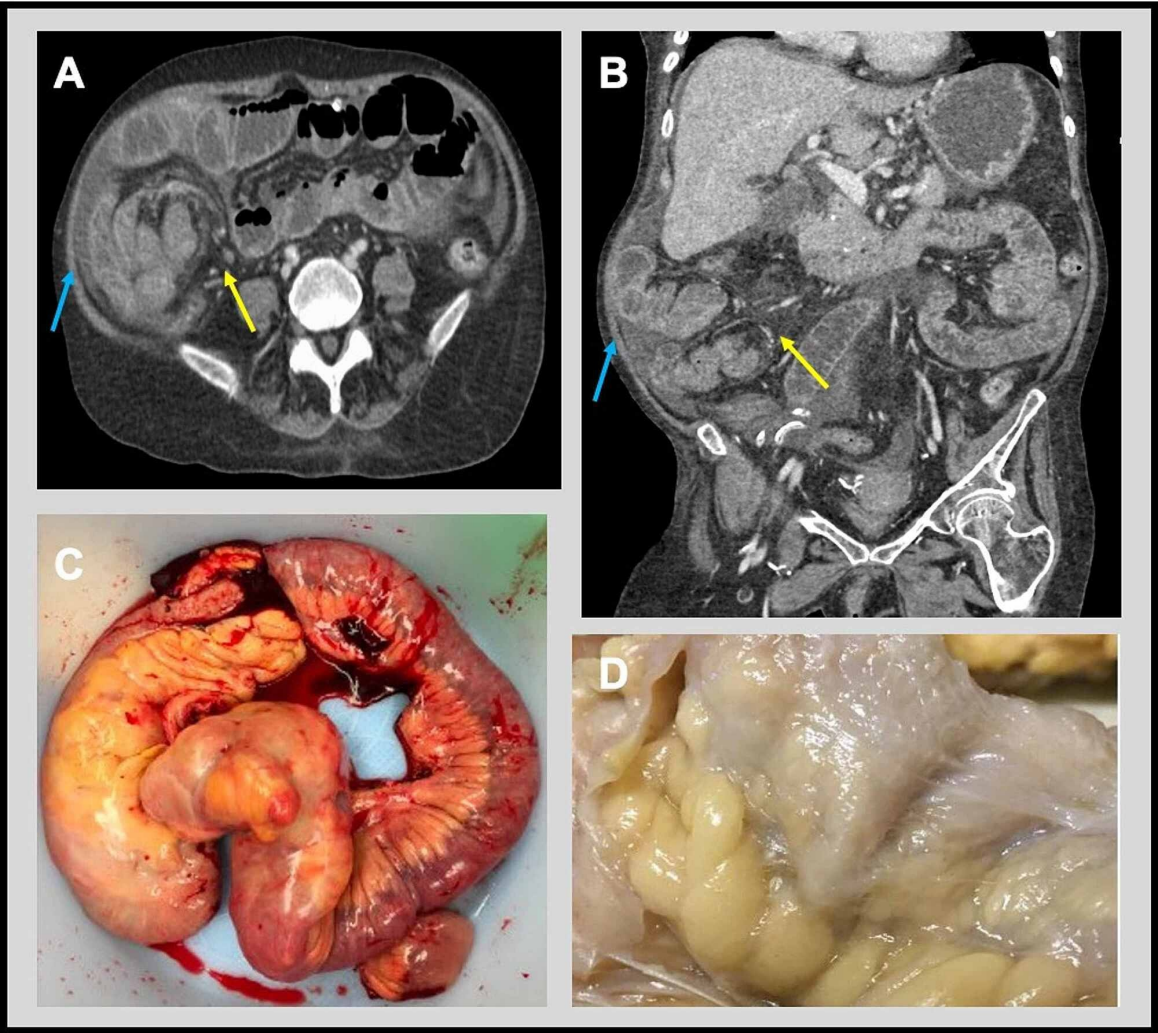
CT and gross pathology findings. CT abdomen/pelvis with IV contrast revealed thickening around the colon, dilatation of small bowel loops possible secondary to small bowel obstruction (blue arrow) with free intraperitoneal air and a transition point in the right lower quadrant (yellow arrow) with findings concerning an internal hernia, as well as pneumatosis in the cecum (A & B). Resection of ileum and right colon with serosa appearing rough in texture with light brown appearance characteristic in EPS (C). The ileum and colon segment has an extensively adhered focus of multiple loops of ileum just proximal to the ileocecal valve (23.0 cm from the proximal margin of resection and 25.0 cm from the distal margin of resection). The adhesion consists a semi-translucent membrane encapsulating the small bowel and its mesentery (D).
